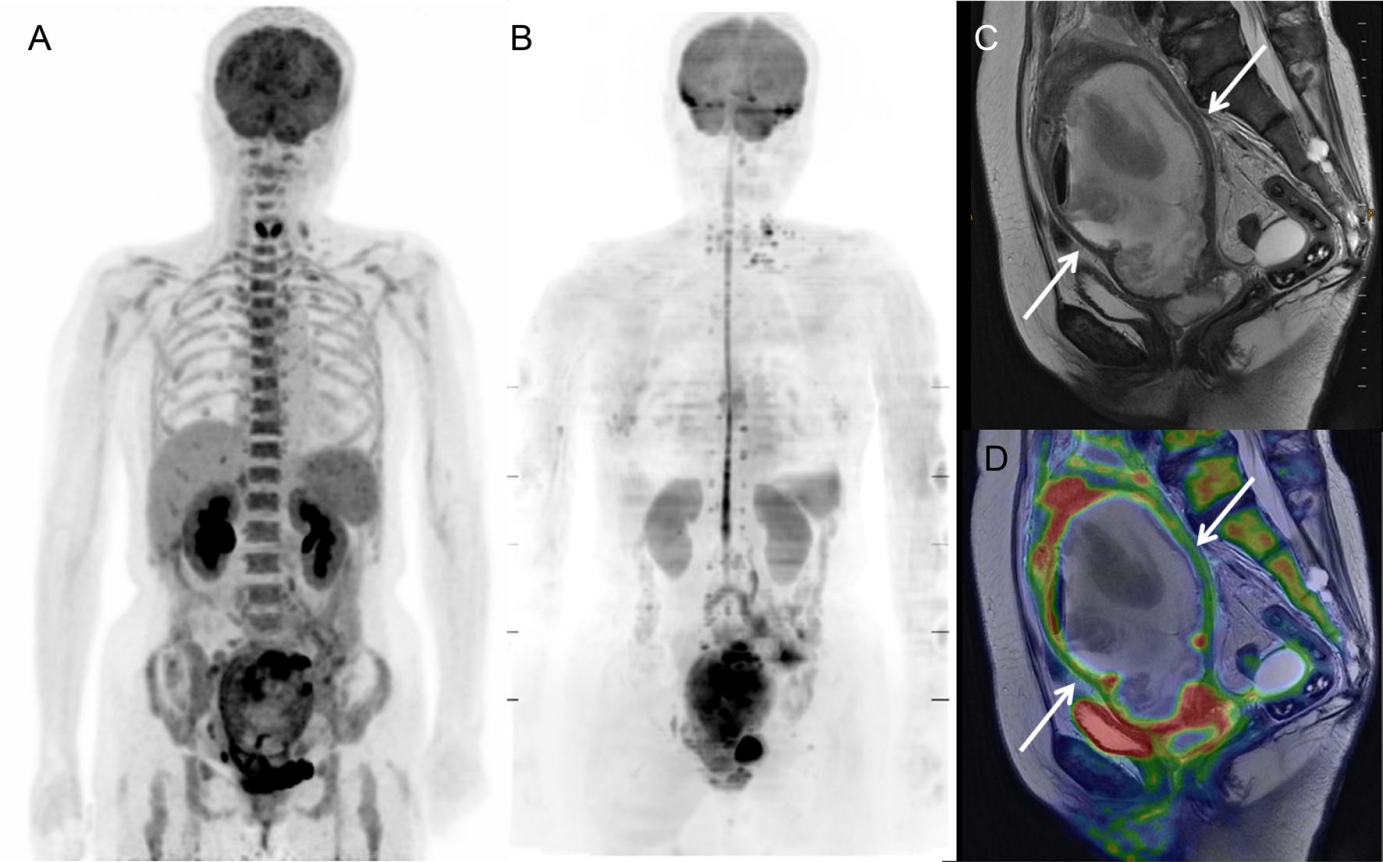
[^18^F]FDG PET and MR images of a 69-year-old woman with uterine cervical cancer and pyometra. Bone marrow exhibited high [^18^F]FDG uptake on PET (lumbar SUV = 3.51 g/mL) (A), whereas it was invisible on DWI with increased R2* (lumbar R2* = 221.3 Hz) (B). Due to obstruction by the primary tumor, severe pyometra was observed on T2-weighted and fusion images (C and D, respectively). Blood data revealed severe inflammation (Hb = 11.1 g/dL, WBC = 11.4 × 10^3^ /μL, and CRP = 22.5 mg/dL).
